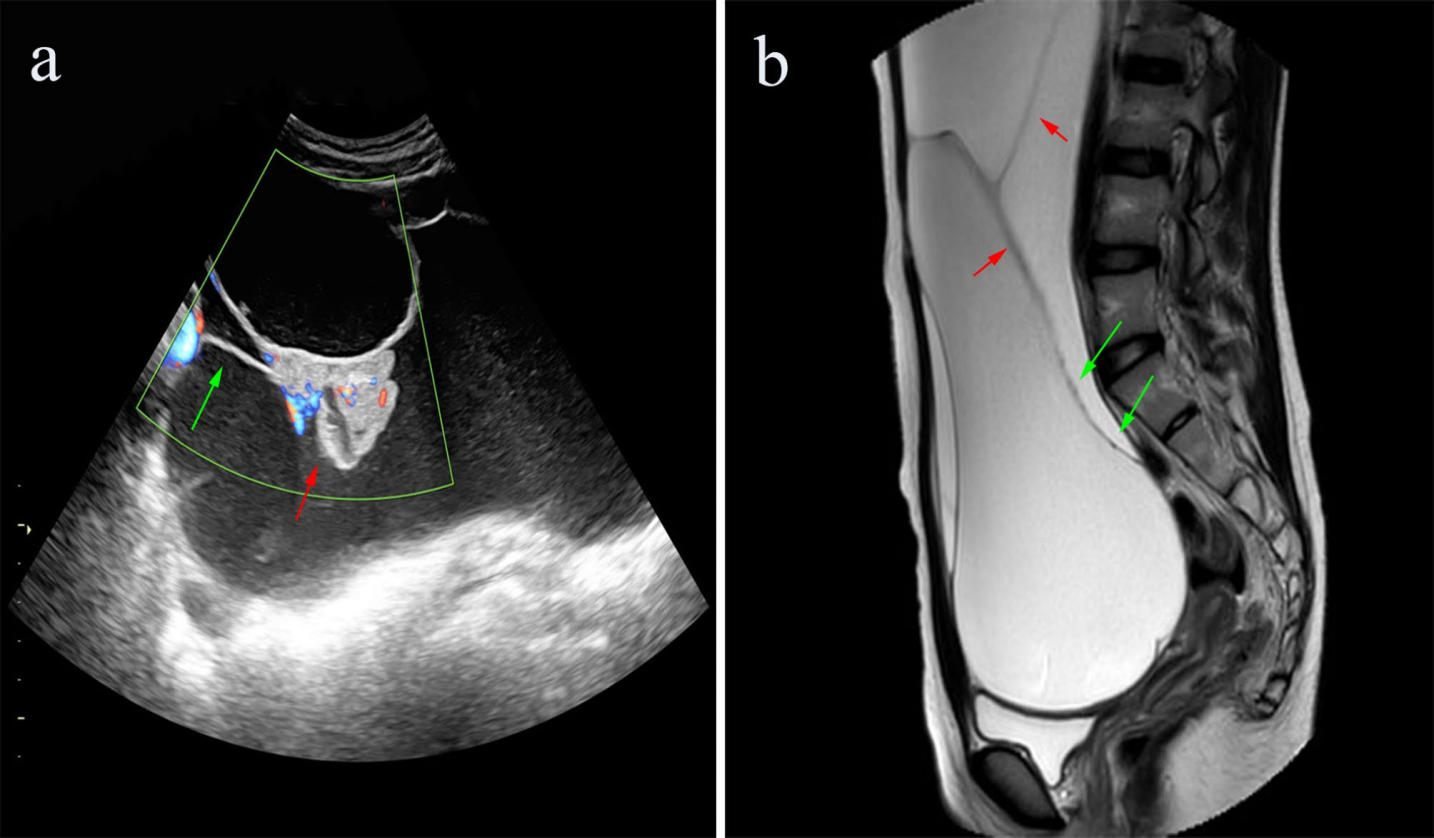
(a) Pelvic color Doppler ultrasound scan revealed a large mass, predominantly cystic, with poor internal echogenicity. It presented as fine, weakly echogenic spots, with numerous unevenly sized septations (green arrow), and several differently sized solid echogenic protrusions into the cystic cavity (red arrow), giving a cauliflower-like appearance. (b) MRI images revealed a large cystic-solid mass, predominantly cystic, with visible septations (red arrow) and wall nodules (green arrow) within
